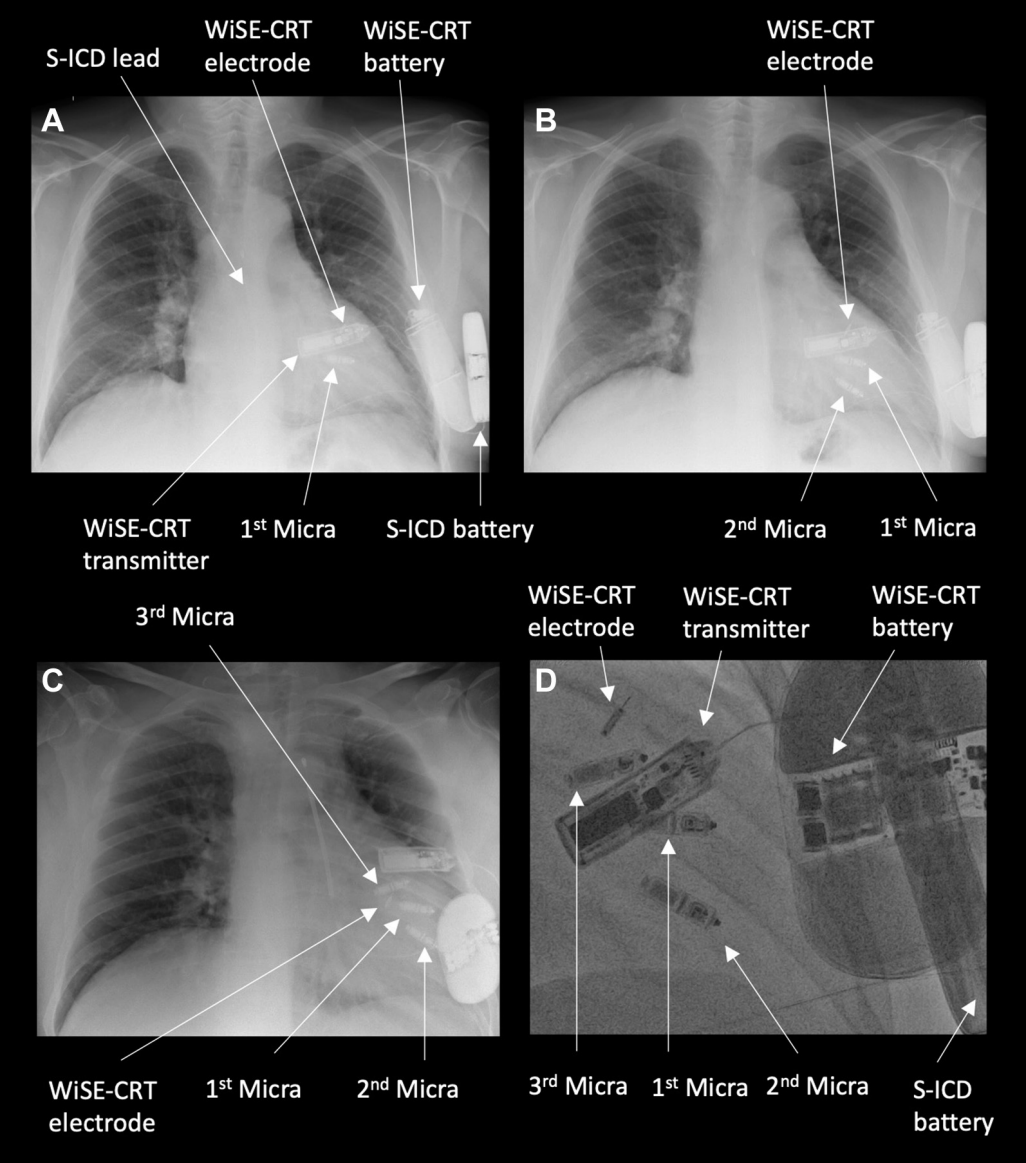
Chest radiographs. A: Completely wireless cardiac resynchronization therapy defibrillator (CRT-D) composed of a Micra leadless pacemaker in the right ventricle, a subcutaneous implantable cardioverter-defibrillator (S-ICD), and the WiSE-CRT system. B: Addition of a second Micra leadless pacemaker inferior to the original implant. C: Addition of a third Micra leadless pacemaker in the right ventricular outflow tract. D: Fluoroscopic right anterior oblique view of completely leadless CRT-D system with 3 Micra leadless pacemakers.
